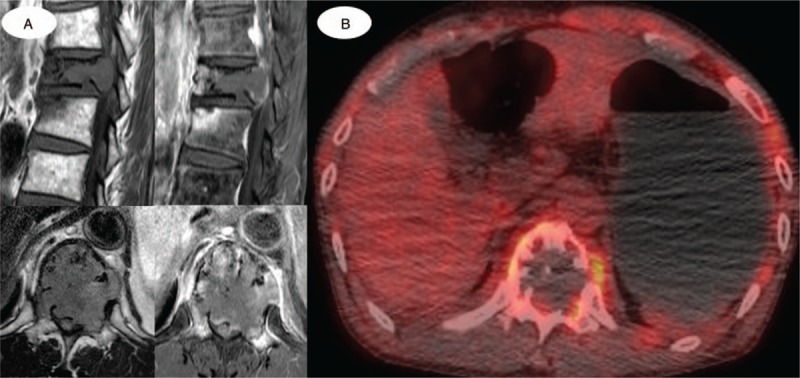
(A) Sagittal and axial MRI images showed the involvement of the entire T12 vertebra and severe canal encroachment, which was highly suspected to be caused by a malignant condition such as metastatic or primary cancer. (B) PET/CT demonstrated a benign condition with a maxSUV of 1.7. CT = computed tomography, PET = positron emission tomography.
